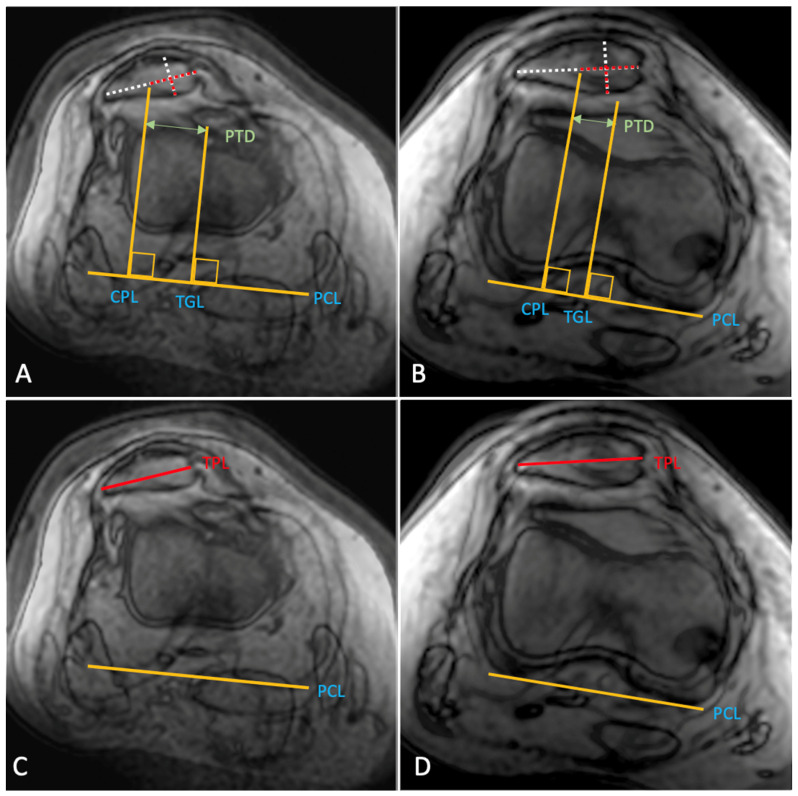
Preoperative and postoperative measurement of the proximal patella position in a fully extended right knee. Compared to the preoperative examination (A), the postoperative patellotrochlear distance (PTD) (B), measured between the deepest point of the trochlea groove (TGL) and the center-center position of the patella (CPL), was significantly reduced. Accordingly, the postoperative patellar tilt (angle between the transpatellar line [TPL] and the posterior condylar line [PCL]) was reduced significantly compared to the preoperative value (C,D). All measurements were performed on transversely oriented MRI slides, in accordance with the technique described by Frings et al. [23].
